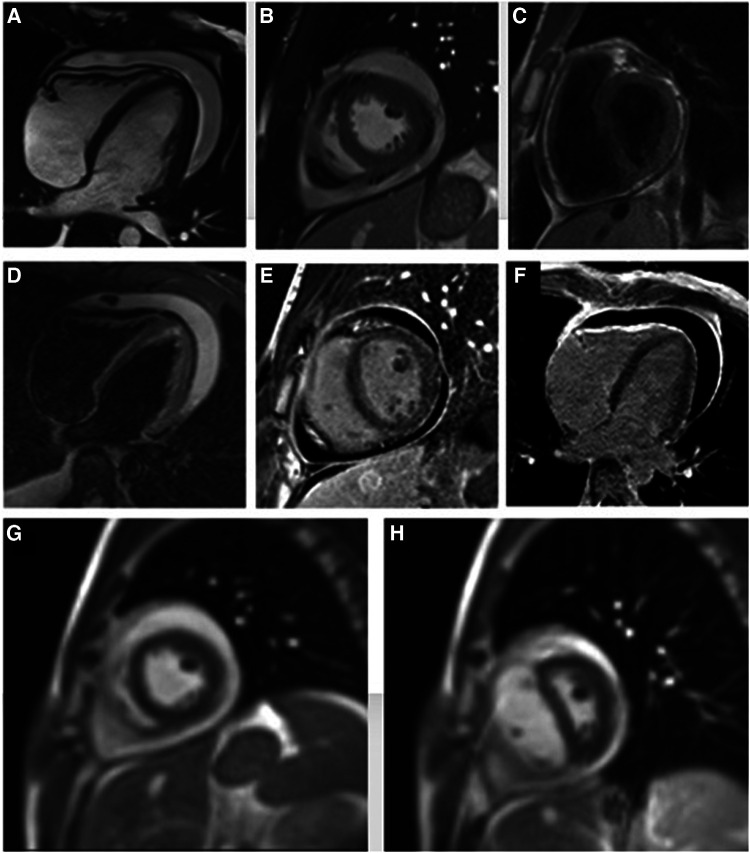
Cine steady-state free precession four- and two-chamber view images show circumferential pericardial effusion (A,B), with uniform pericardial thickening at T1-weighted short-axis view image (C). There is no significant hyperintense signal on the edema-weighted image (D) while a marked late gadolinium enhancement of pericardial layers is evident (E,F), suggesting diffuse pericardial fibrosis. Short-axis real-time cine MRI sequence during operator-guided breathing (G,H) demonstrates an abnormal displacement of the interventricular septum during the onset of inspiration, due to a fixed pericardial volume with reduced ventricular compliance and consequent increase of the right ventricular filling on inspiration.
